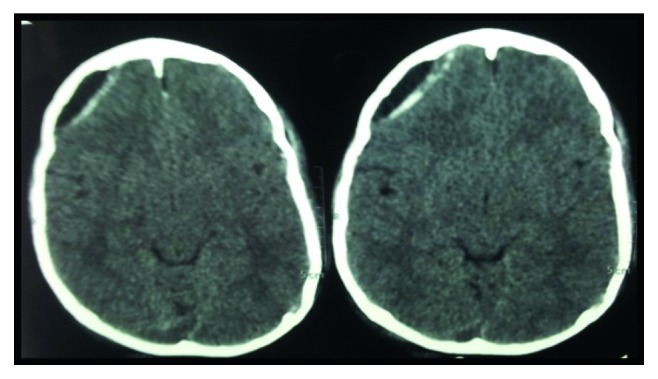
Postoperative CT scan showing subdural hemorrhage in temporoparietal region.
